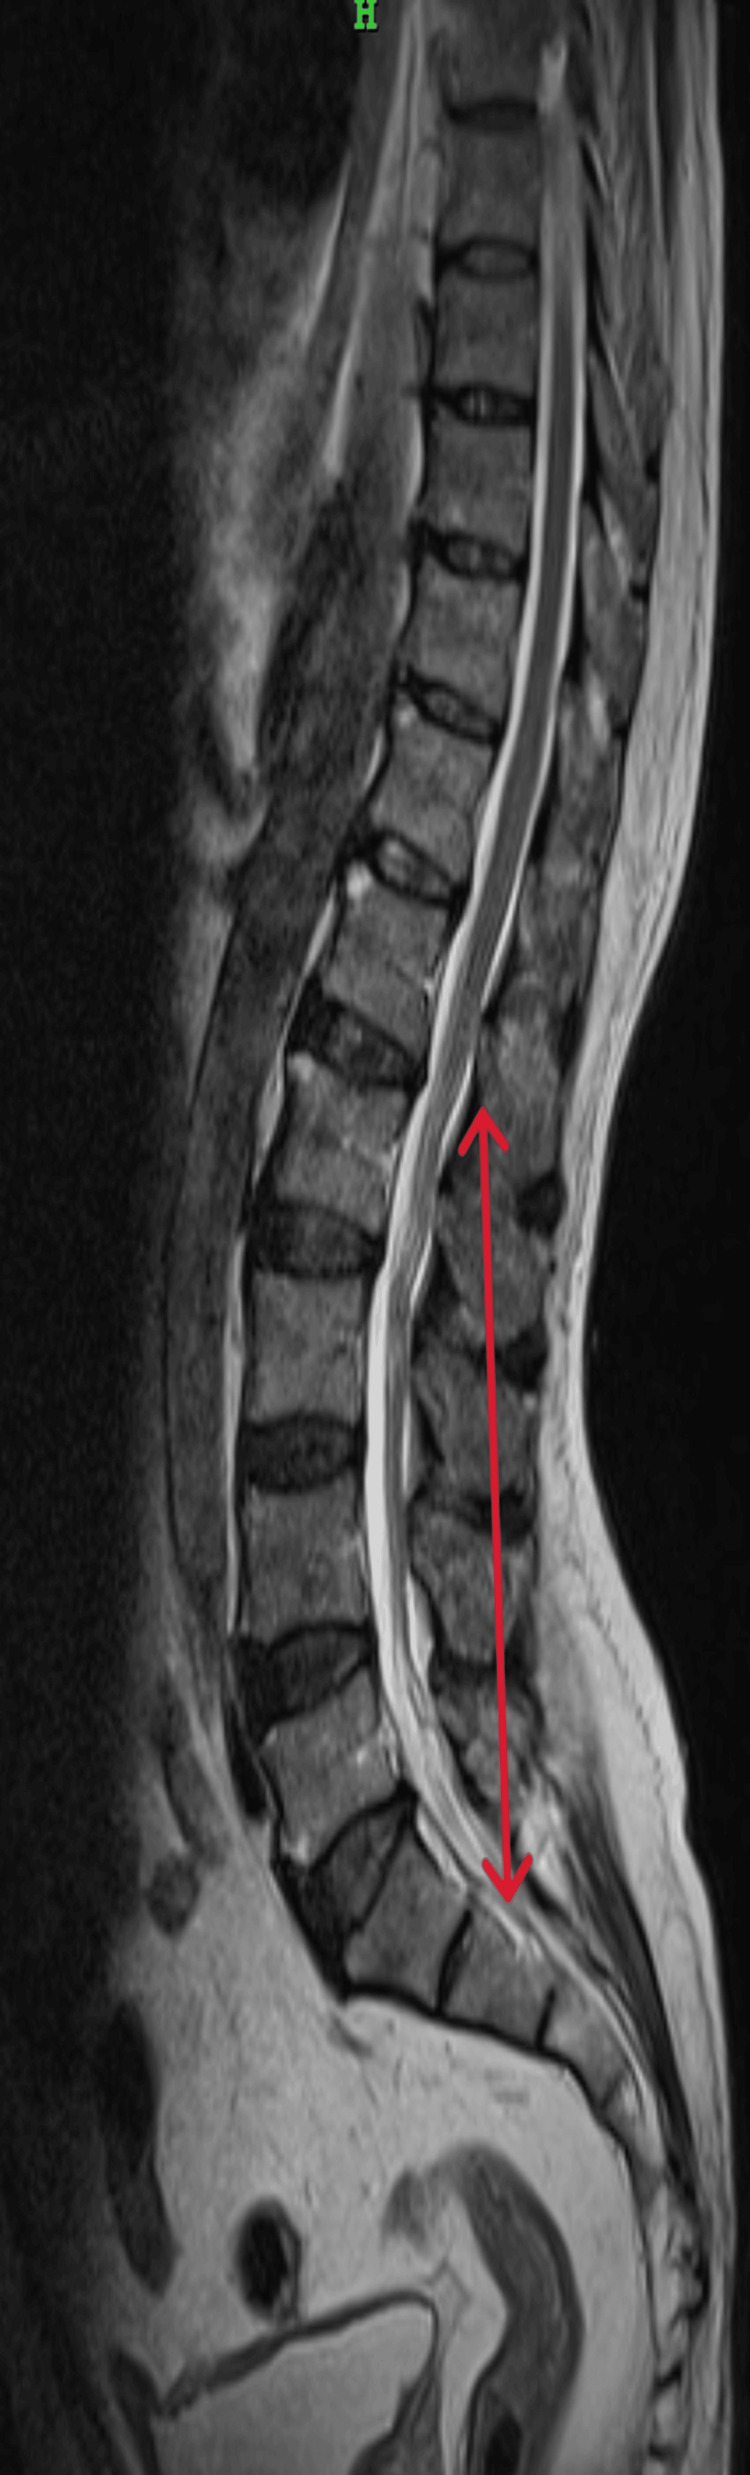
Magnetic resonance imaging of lumbar spine (axial view) Red arrow indicates posterior disc bulges at L2-L3, L3-L4, L4-L5, L5-S1.
